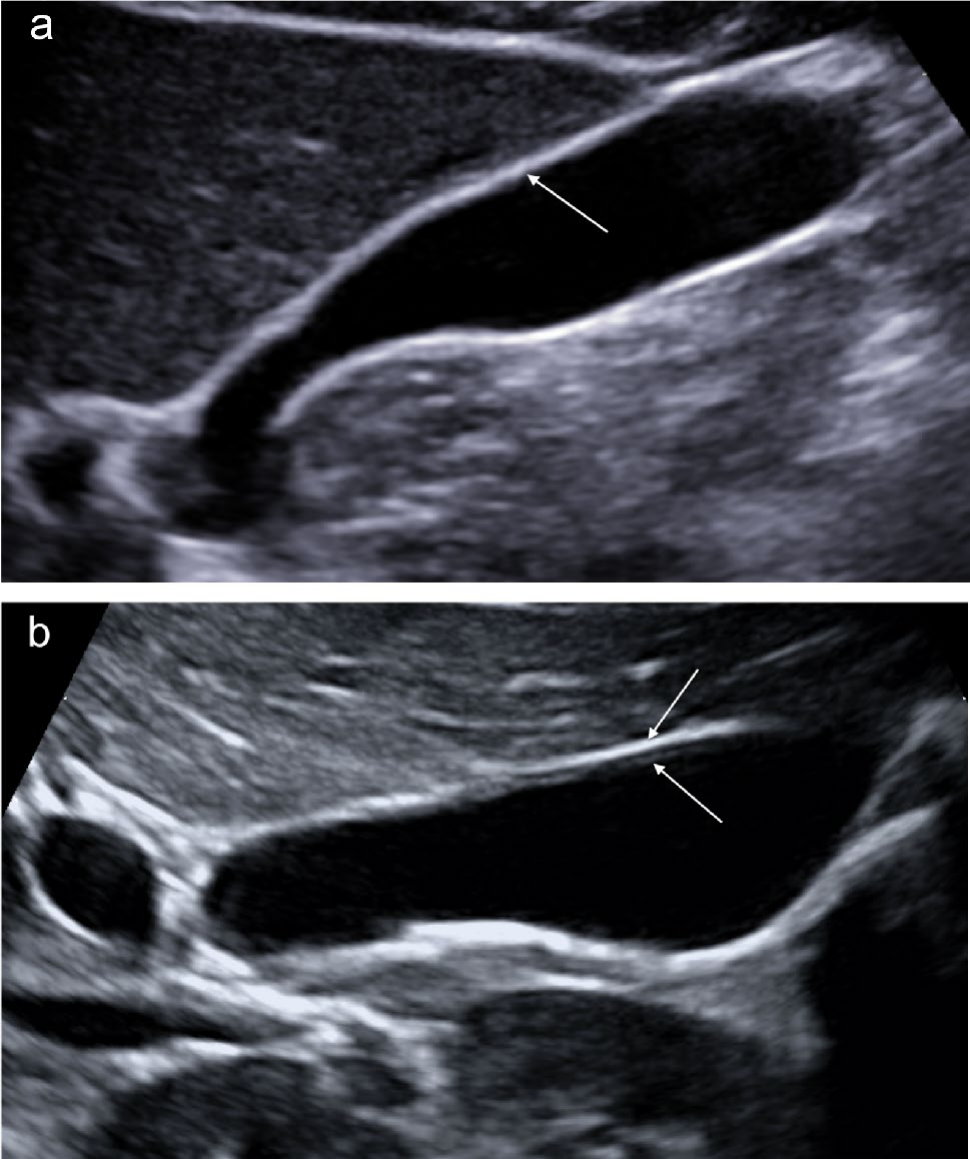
a Conventional transabdominal sonography image using a convex probe (1–5 MHz) shows a single layer (white arrow). b Conventional transabdominal sonography image using a convex probe (1–5 MHz) shows two layers of the gallbladder wall (white arrows)
